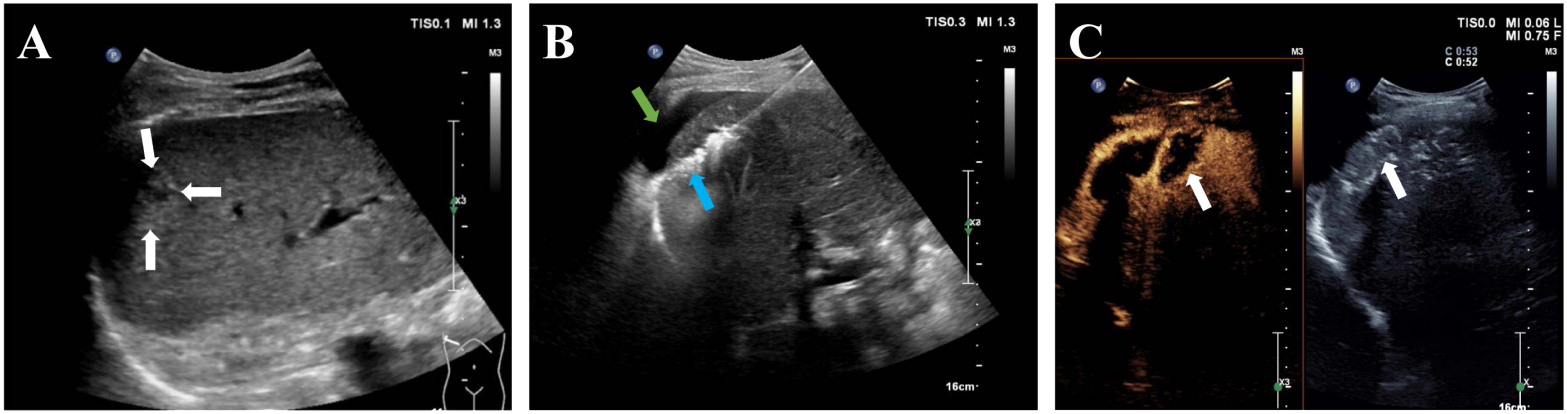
A 68-year-old man with hepatocellular carcinoma before and after MWA. (A) Ultrasound image showed a 1.6-cm hepatocellular carcinoma in the subcapsular region of the liver (white arrow).; (B) After the saline injection hydrodissection (green arrow), the electrode needle was inserted into the tumor under ultrasound guidance (blue arrow); (C) Postoperative CEUS showed no enhancement in the whole ablation area (white arrow).
